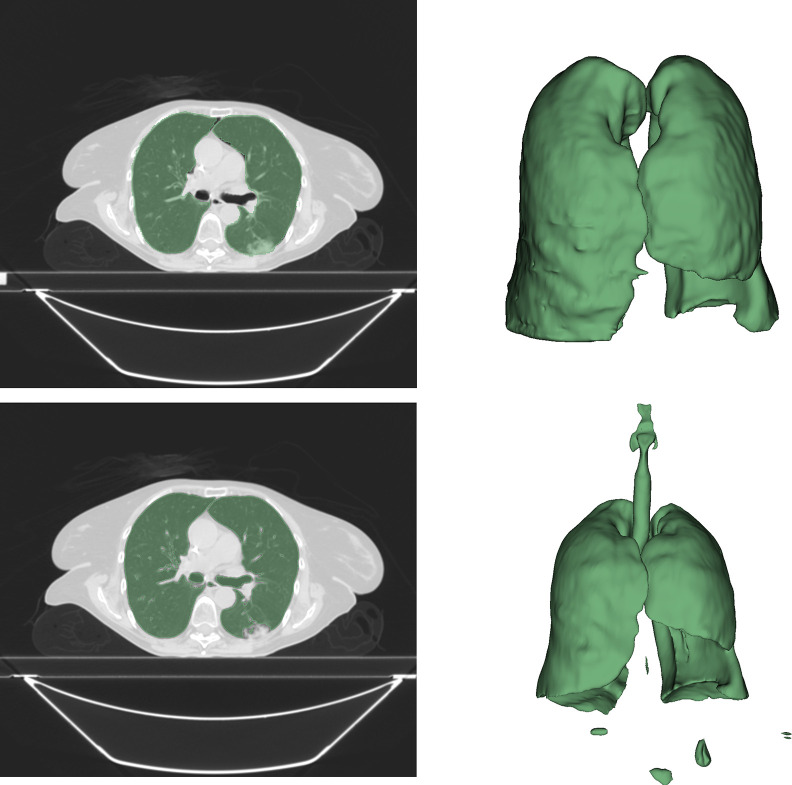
CT images of the lung with segmentation (shown in green). The top left
